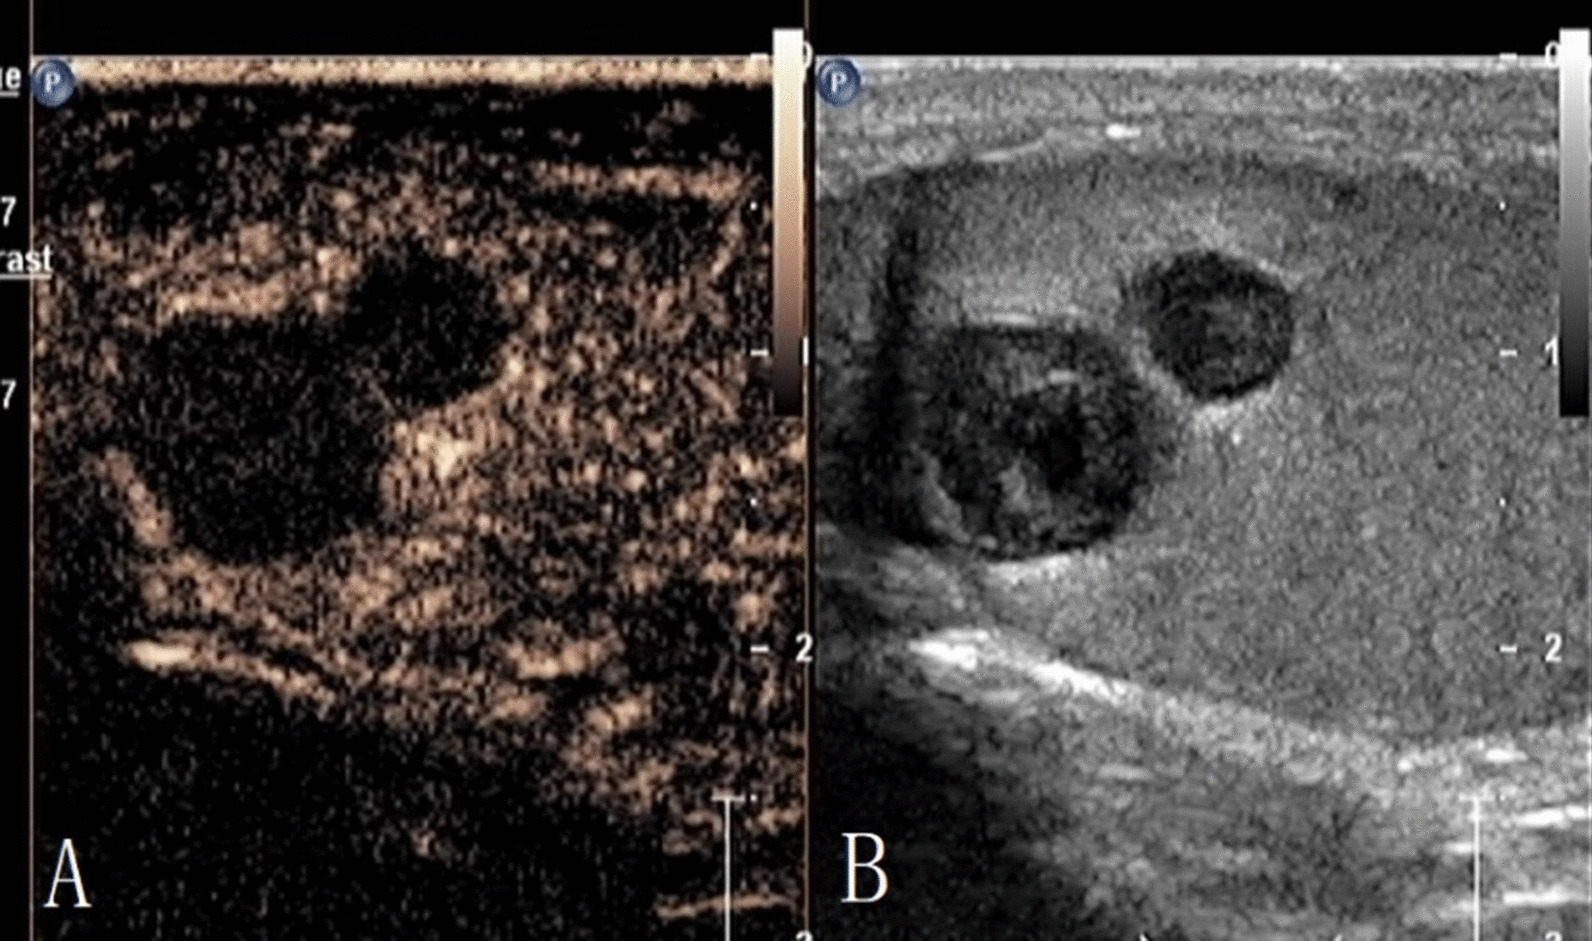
Testicular spontaneous hematoma. A Contrast-enhanced ultrasonography showed no enhancement in the mass and a diagnosis of spontaneous hematoma was made. B Conventional ultrasound showed that the right testis had clear boundary and irregular hypoecho. Because there was no history of trauma, this was considered to be malignant tumor
